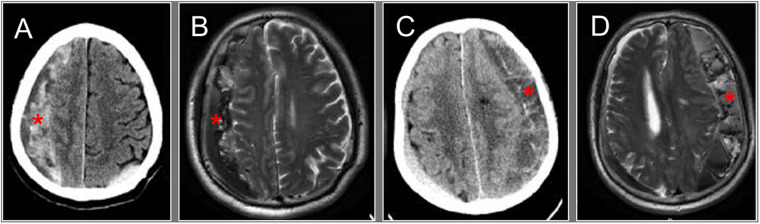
The preoperative cranial CT scan (A and C) and MRI (B and D) from two patients. Images A, B and C, D are from the same patient respectively. The asterisks in A-D indicate the locations of the subdural hematoma presented as mixed-density or heterogeneous signal intensity. This radiographic feature suggests the possible presence of fibrous membrane septa and residual blood clots within the hematoma cavity. Generally, the more heterogeneous the density of the hematoma, the more complex the structure of the intracavitary fibrous membranes tends to be. Intraoperatively, with the assistance of a endoscope, these fibrous membranes can be directly observed diffusely distributed throughout the hematoma cavity, which impedes the placement of drainage tubes and irrigation of the cavity. This constitutes a key factor making complete evacuation of the hematoma particularly challenging.
